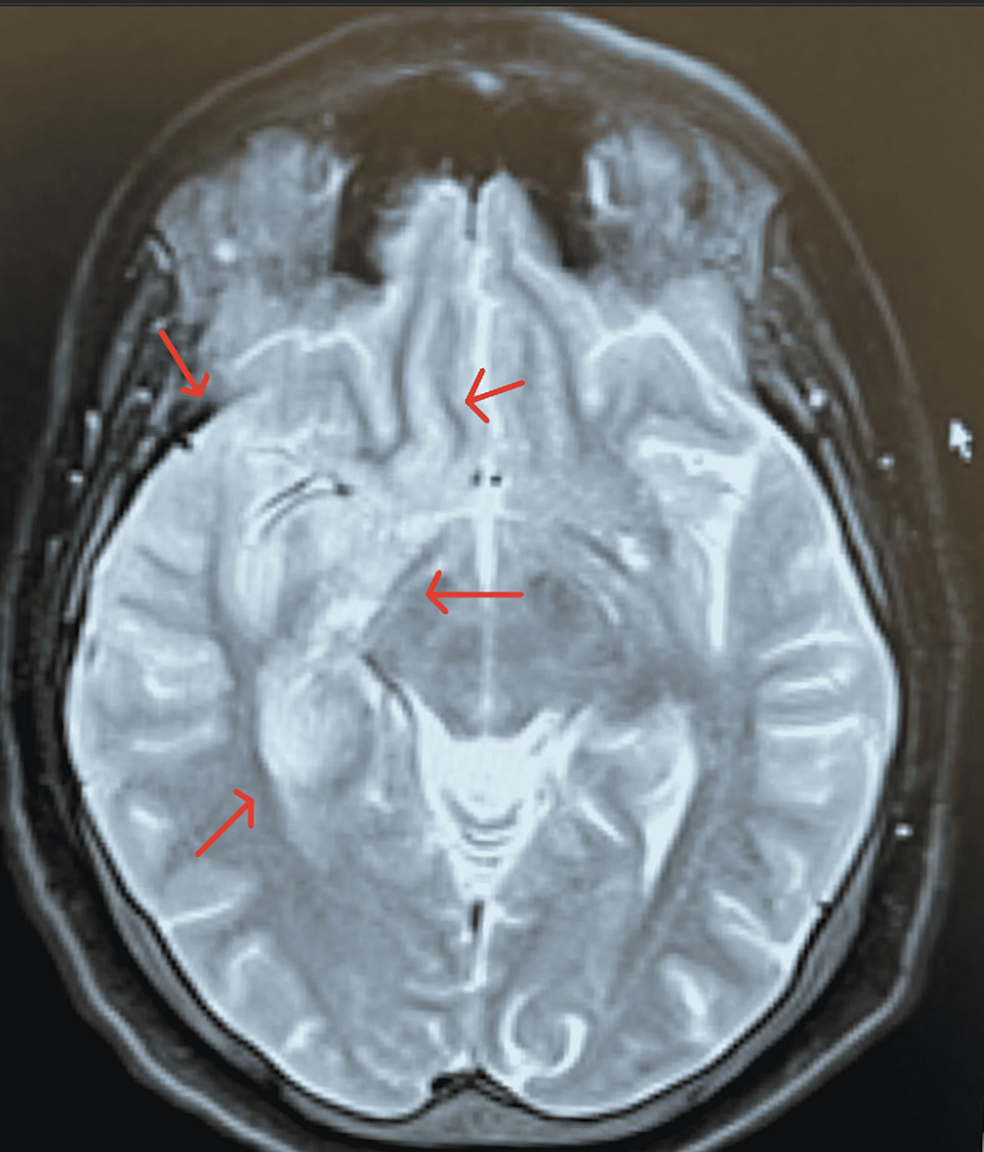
MRI of the brain with contrast in a T2-weighted axial view The axial view shows increased signal intensity and diffusion restriction is seen in the anteromedial aspect of the right temporal lobe, insular cortex, and right hippocampus (red arrows). There is a loss of gray-white differentiation in the medial aspect of the right temporal lobe.
